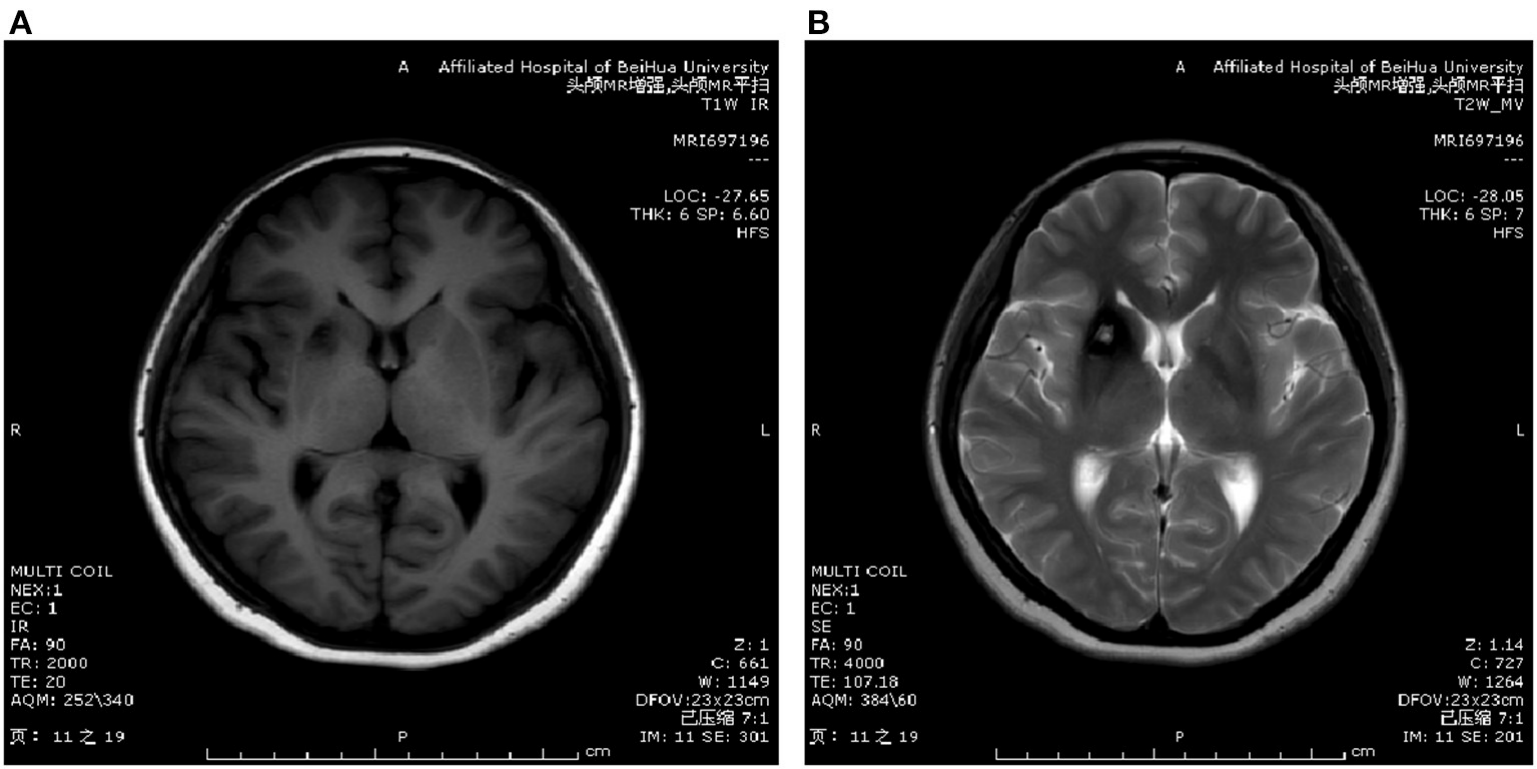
MRI scanning image. (A) Shows T1WI image and (B) shows T2WI image.
